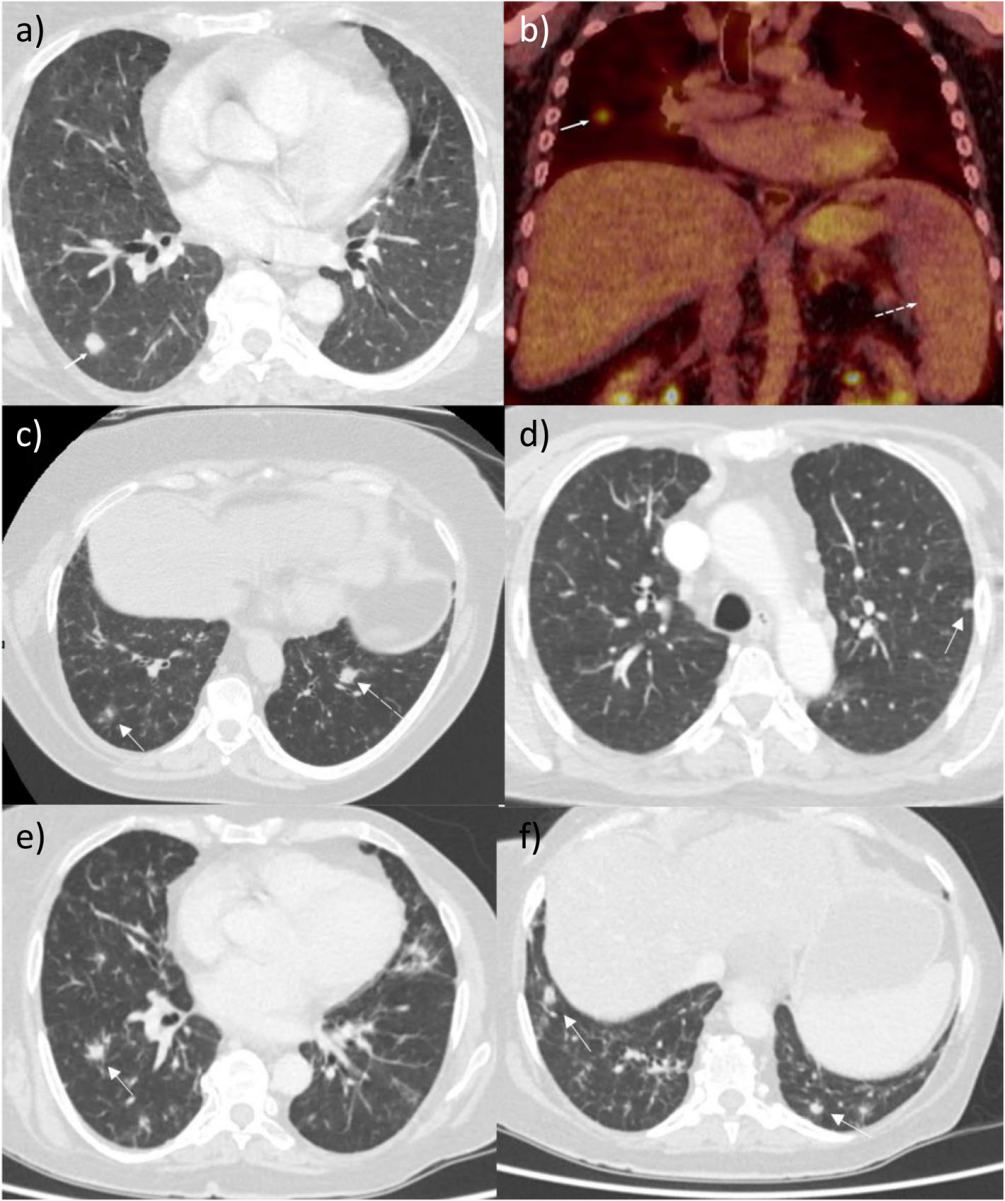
CT and PET imaging. a CT Thorax showing a right lower lobe pulmonary nodule (arrow, June 2016), b PET-CT showing the same right lower lobe pulmonary nodule (arrow) and splenomegaly (broken arrow). c New left lower lobe pulmonary nodule (broken arrow) and resolving right lower lobe nodule (arrow, July 2016). d New left lower lobe nodule (arrow, April 2018) e New right lower lobe nodule (arrow, Sept 2019) f) New lower lobe nodules (arrows, Sept 2019)
